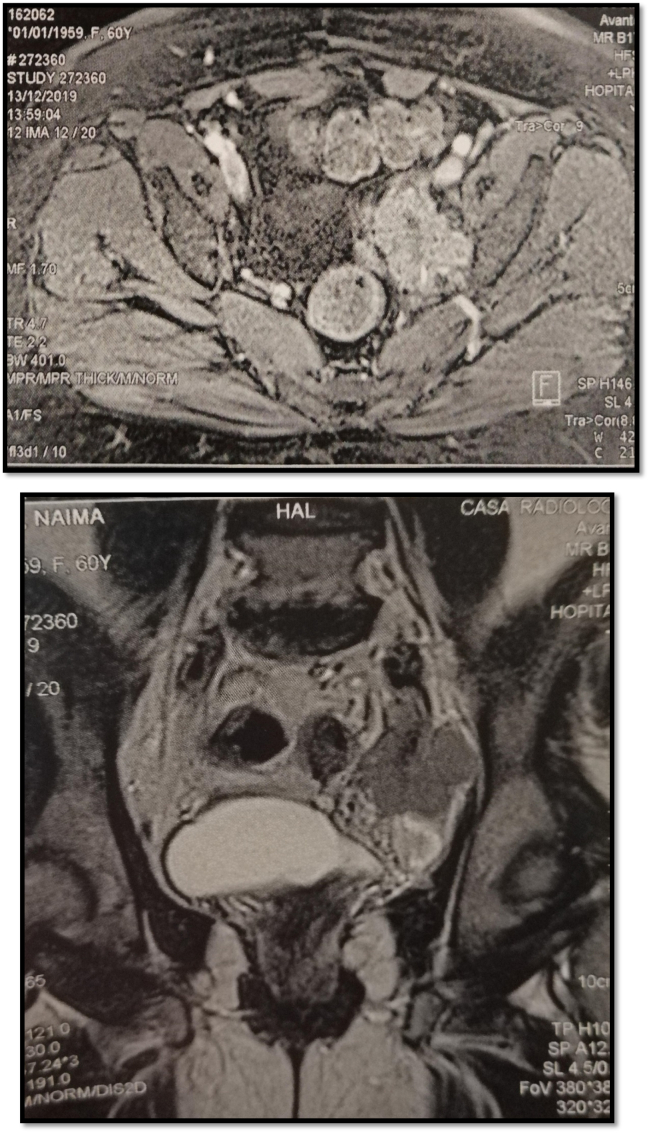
MRI appearance of recurrent tubal adenocarcinoma.
